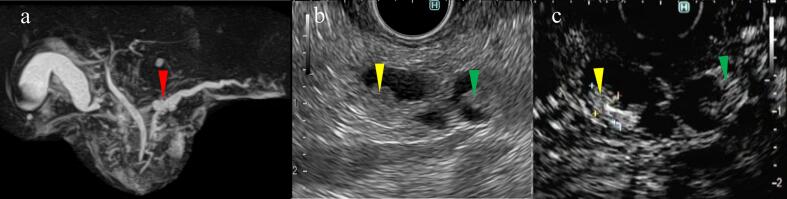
a: Magnetic resonance imaging findings reveal complete main pancreatic duct dilatation, and a cystic lesion (red arrowhead) with a septal wall is situated in the cephalic transition region, making direct contact with the main pancreatic duct. b: Endoscopic ultrasonography findings showing nodules measuring 6 mm in the main pancreatic duct (yellow arrowhead) and a cyst (green arrowhead). c: The nodules (arrowheads) were contrast-enhanced with Sonazoid. (For interpretation of the references to colour in this figure legend, the reader is referred to the web version of this article.)
